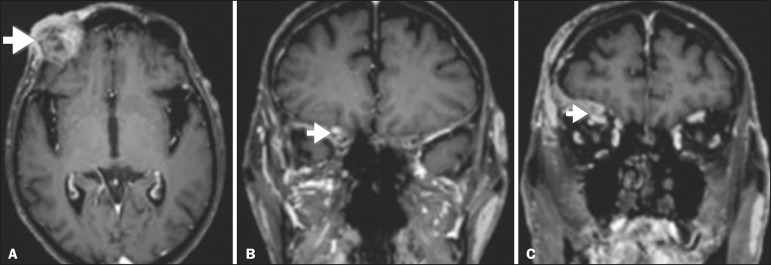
Contrast-enhanced T1-weighted MRI scans of a 83-year-old male patient
